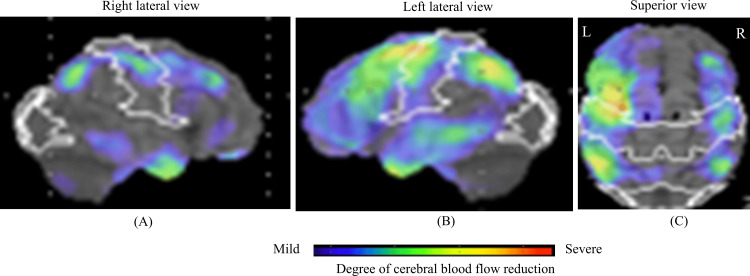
Single-photon emission computed tomography (SPECT) findings Cerebral blood flow (CBF) reduction visualized from three perspectives using 3D surface projections. The color scale indicates the degree of CBF reduction, ranging from mild (blue) to severe (red). (A) Right lateral view showing mild-to-moderate hypoperfusion in the right frontal and parietal cortices. (B) Left lateral view demonstrating more extensive hypoperfusion in the left frontal, temporal, and parietal regions. (C) Superior view highlighting bilateral but asymmetric hypoperfusion, predominantly in the left hemisphere.
